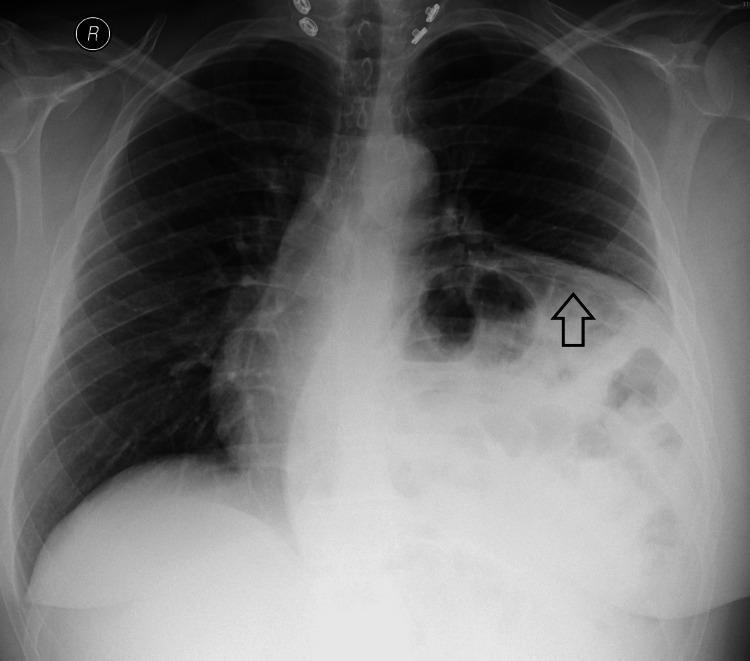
Chest x-ray showing elevated left hemidiaphragm.
